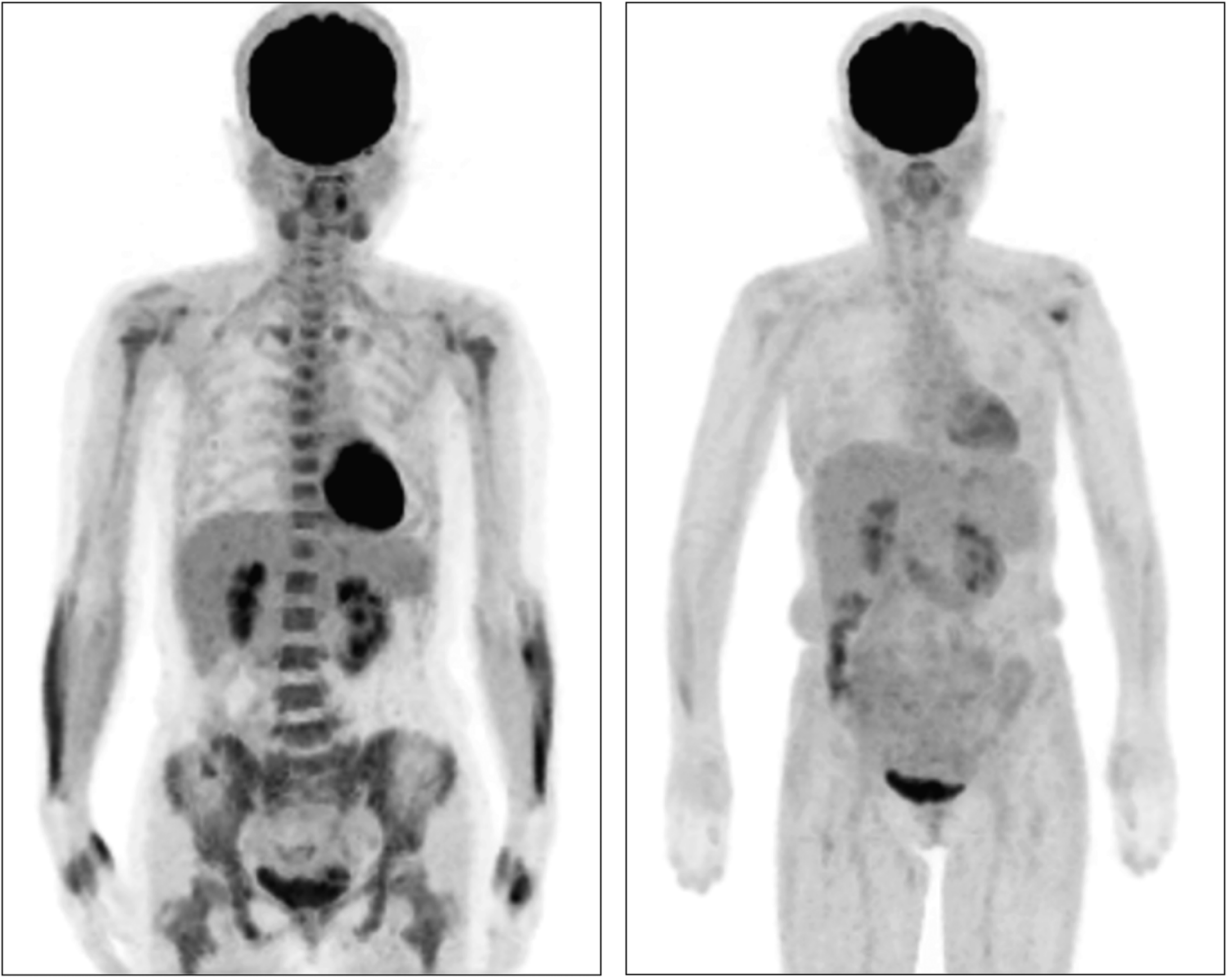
The left image shows increased FDG uptake throughout the bone marrow as demonstrated by pretreatment PET‐CT. The right image presents posttransplantation PET/CT imaging, confirming the resolution of the previously noted anomalous FDG accumulation. FDG, fluorodeoxyglucose; PET/CT, positron emission tomography/computed tomography.
